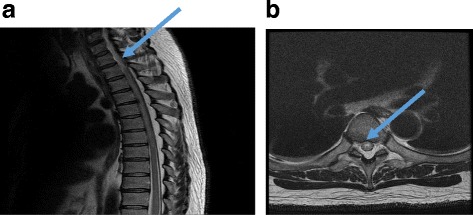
a Sagittal T2-weighted magnetic resonance imaging (MRI) scan through the thoracic spinal cord obtained on postoperative day 2 showing abnormal T2 high signal intensity from the Th3 to Th8 levels (arrow). b Axial T2-weighted image showing high signal intensity in the anterior horn of the gray matter (owl’s eye) at the Th5 level (arrow)
